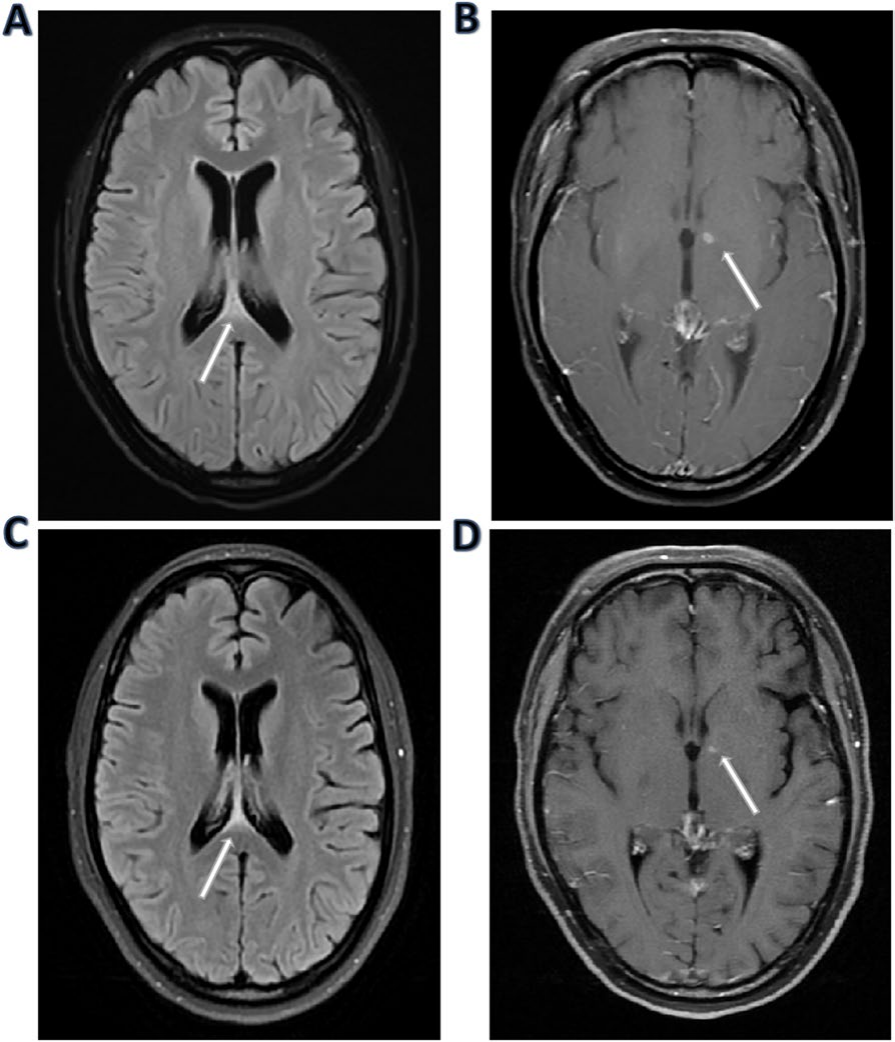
Brain MRI results. A A few enhancement lesions on the upper edge of the cerebellar tonsil. B The dominant enhancement in the left basal ganglia is probably infection foci. C, D A decrease in the upper edge of the cerebellar tonsil and left basal ganglia lesion size
